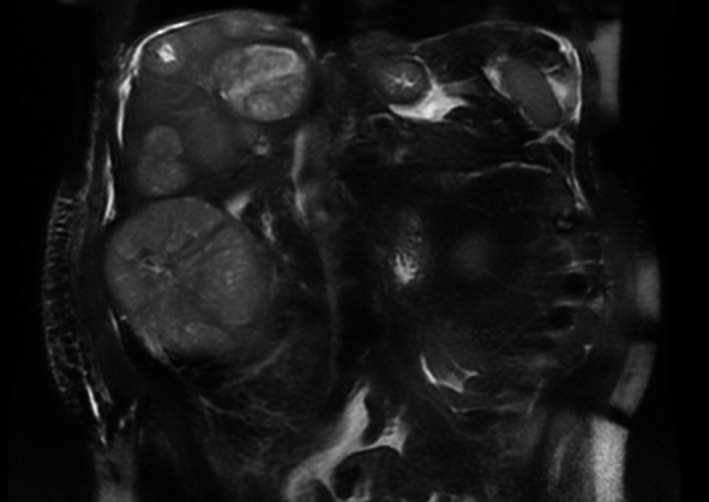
Multifocal Liver Lesions on MRI Abdomen with and without Contrast, T2 Weighted Image
